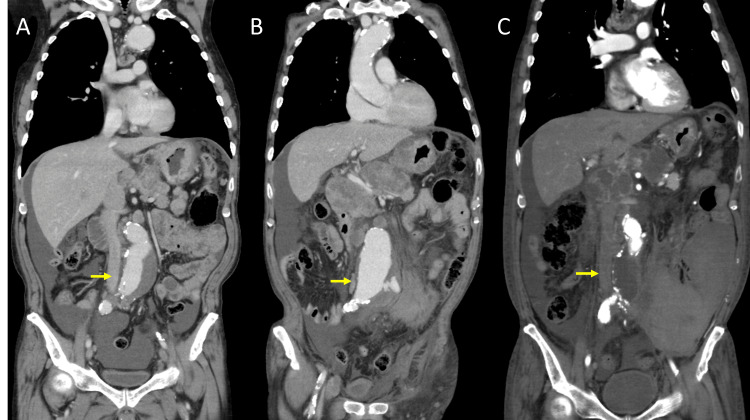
Comparison of pre-treatment and post-rupture CT findings. (A) Pre-treatment coronal CT image showing abdominal aortic aneurysm. (B) Coronal CT image after rupture demonstrating an enlarged aneurysm measuring 70 mm. (C) Postoperative coronal CT image showing a 22×11 mm aorto-uni-iliac Dacron graft, consistent with that shown in Figure 1D. Arrows in each panel indicate the location of the aortic aneurysm or the aortic graft.
